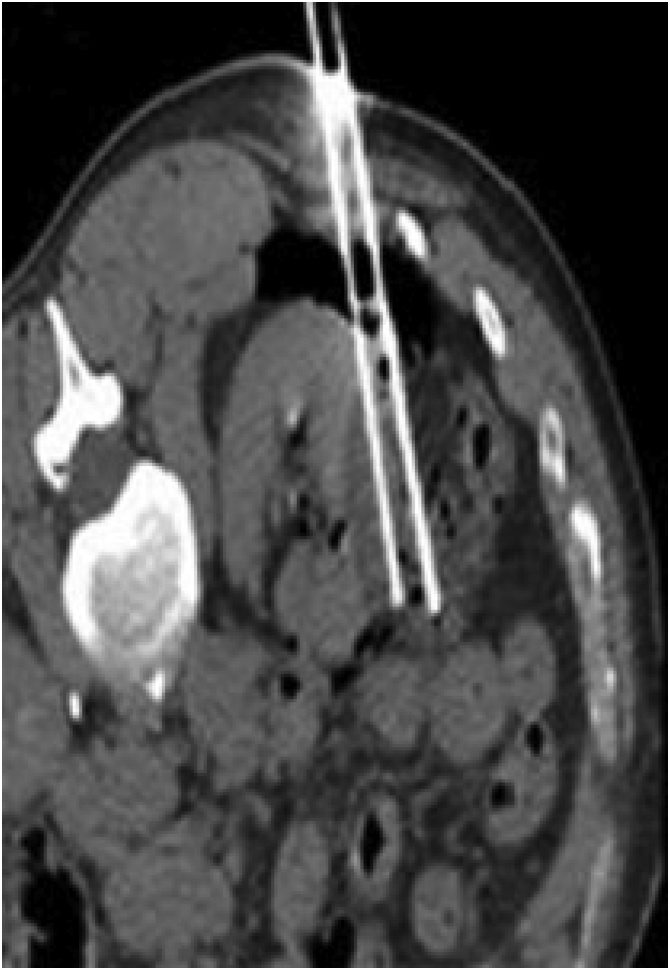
Percutaneous cryoablation monitoring of the cortical tumour at the lower pole of the left kidney using computed tomography.
